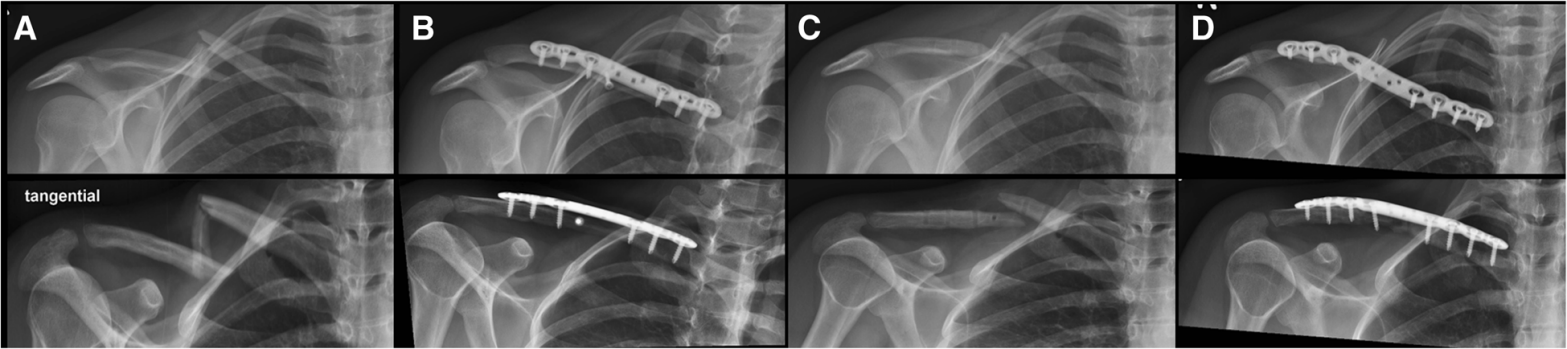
a X-ray in two planes of a 32 years old female patient with multifragmentary midshaft clavicular fracture, b 5 months after surgery with consolidation of the fracture and c 5 days after hardware removal done 14 months after initial surgery showing refracture after minor trauma and d 6 weeks after revision surgery with a VariAx locking plate
